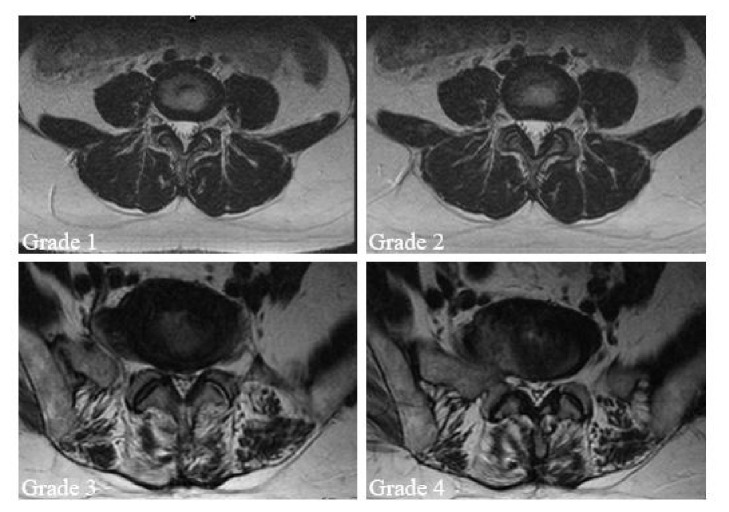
Facet osteoarthritis changes or degeneration was categorized into four grades using T2-weighted axial MRI. (a) Grade 1 was normal. (b) Grade 2 showed a narrow joint space and small osteophytes (mild osteoarthritis). (c) Grade 3 showed sclerosis or subchondral erosions (moderate osteoarthritis). (d) Grade 4 showed marked osteophyte (severe osteoarthritis).
